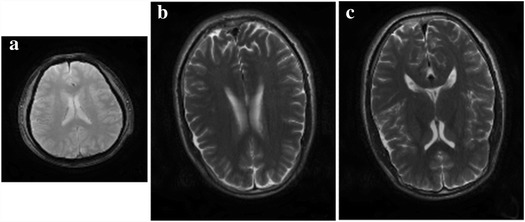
Input images. The original HR T2-weighted vision of (a) is acquired on a 3 T Philips scanner; Original HR T2-weighted visions of (b) and (c) are acquired on a 3 T SIMENS scanner
